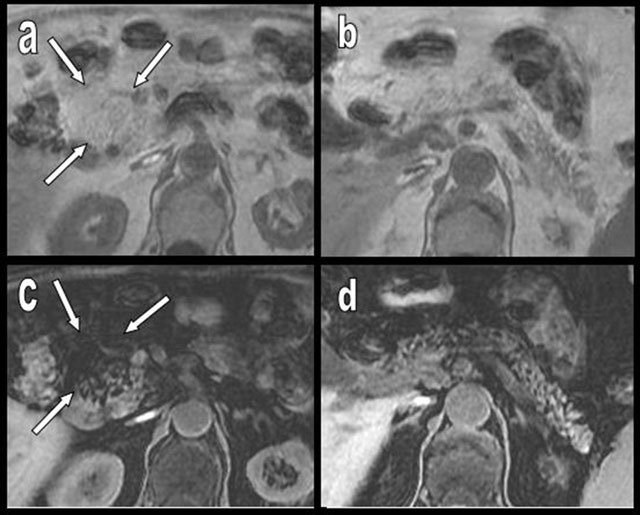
Typical MRI aspects in a case of diffuse FI of the pancreas. FI predominates on the APH (type 1a) with glandular enlargement (white arrows). On T1-weighted images (a and b), the lipomatous APH has a typical high signal. Drastic extinction of the signal is found on T1-weighted images with fat suppression (c and d).
